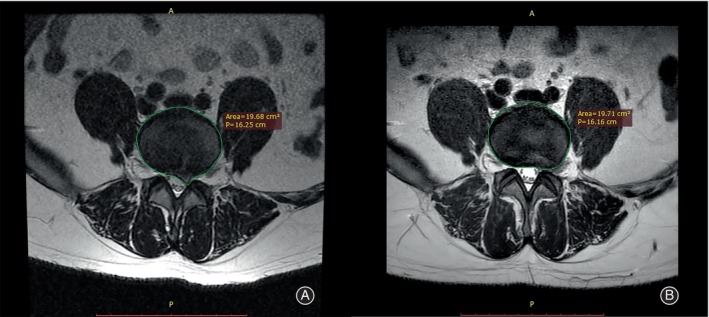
A 39‐year‐old patient is shown preoperatively and postoperatively at the same lumbar spine MRI cross‐section level. The disc area preoperatively was 19.68 cm^2^ and postoperatively was 19.71 cm^2^. The changes in VAS‐B at 3, 6, and 12 months postoperatively were 3, 3, and 4. Those in VAS‐L at 3, 6, and 12 months postoperatively were 6, 6, and 7.
